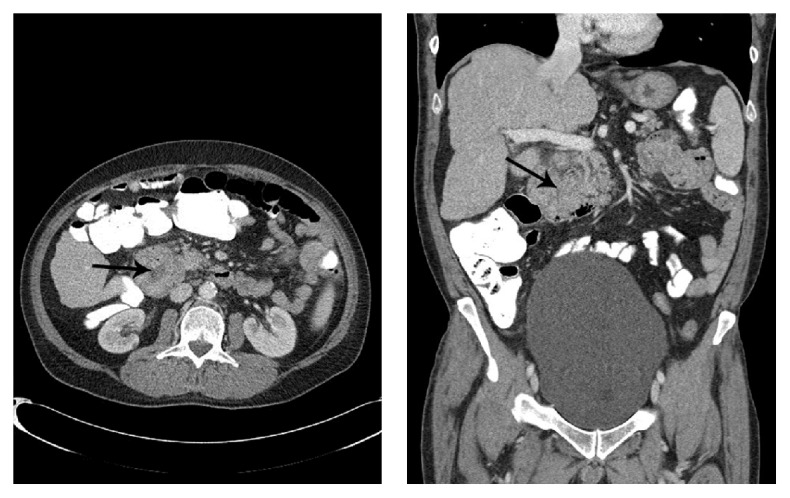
Axial and coronal CT of the abdomen demonstrating a 4.7 cm heterogeneously enhancing mass (arrow) involving the second portion of the duodenum. The lumen of the duodenum is compressed by the mass.
